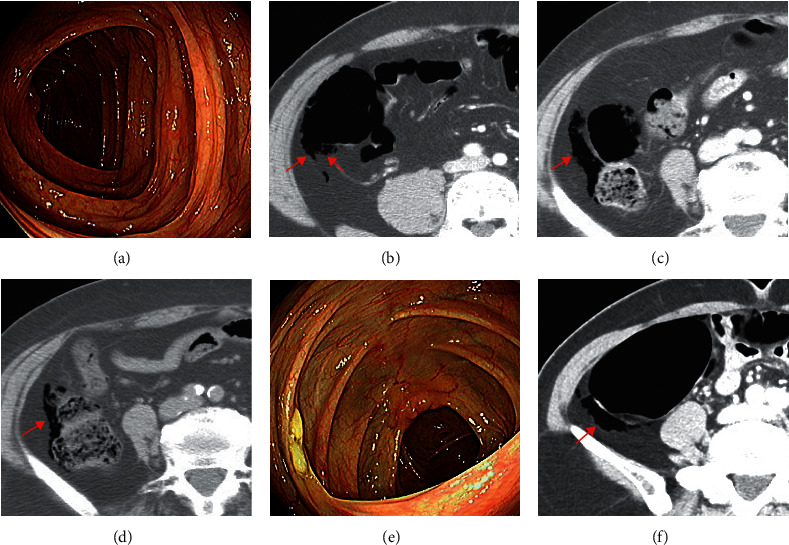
(a) Normal colonoscopic findings in case 1. (b) Abdominal computed tomography (CT) shows a small amount of free air in the right paracolic gutter in case 1. (c) Abdominal CT shows a small amount of free air in the right paracolic gutter in case 2. (d) Follow-up CT scan shows a reduced pneumoperitoneum two months after its initial detection in case 2. (e) Normal colonoscopic findings in case 3. (f) Abdominal CT shows a small amount of free air in the right paracolic gutter in case 3.
